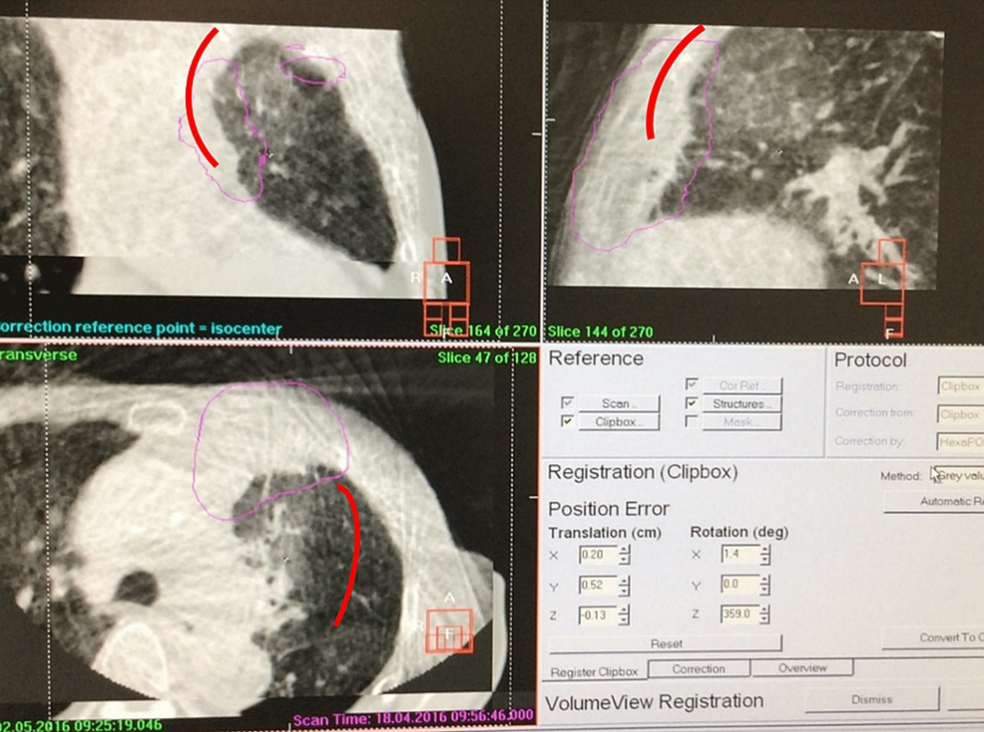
CBCT images in three orthogonal planes with a lung window setting RP is suggested by the red lines. On each of the previous treatment days, a mediastinal window setting was employed for tumor registration, and the window setting was changed to lung because the patient showed RP symptoms. Our retrospective investigation revealed that we could have observed RP three days earlier if the lung window setting had been employed. CBCT - cone-beam computed tomography; RP - radiation pneumonitis
